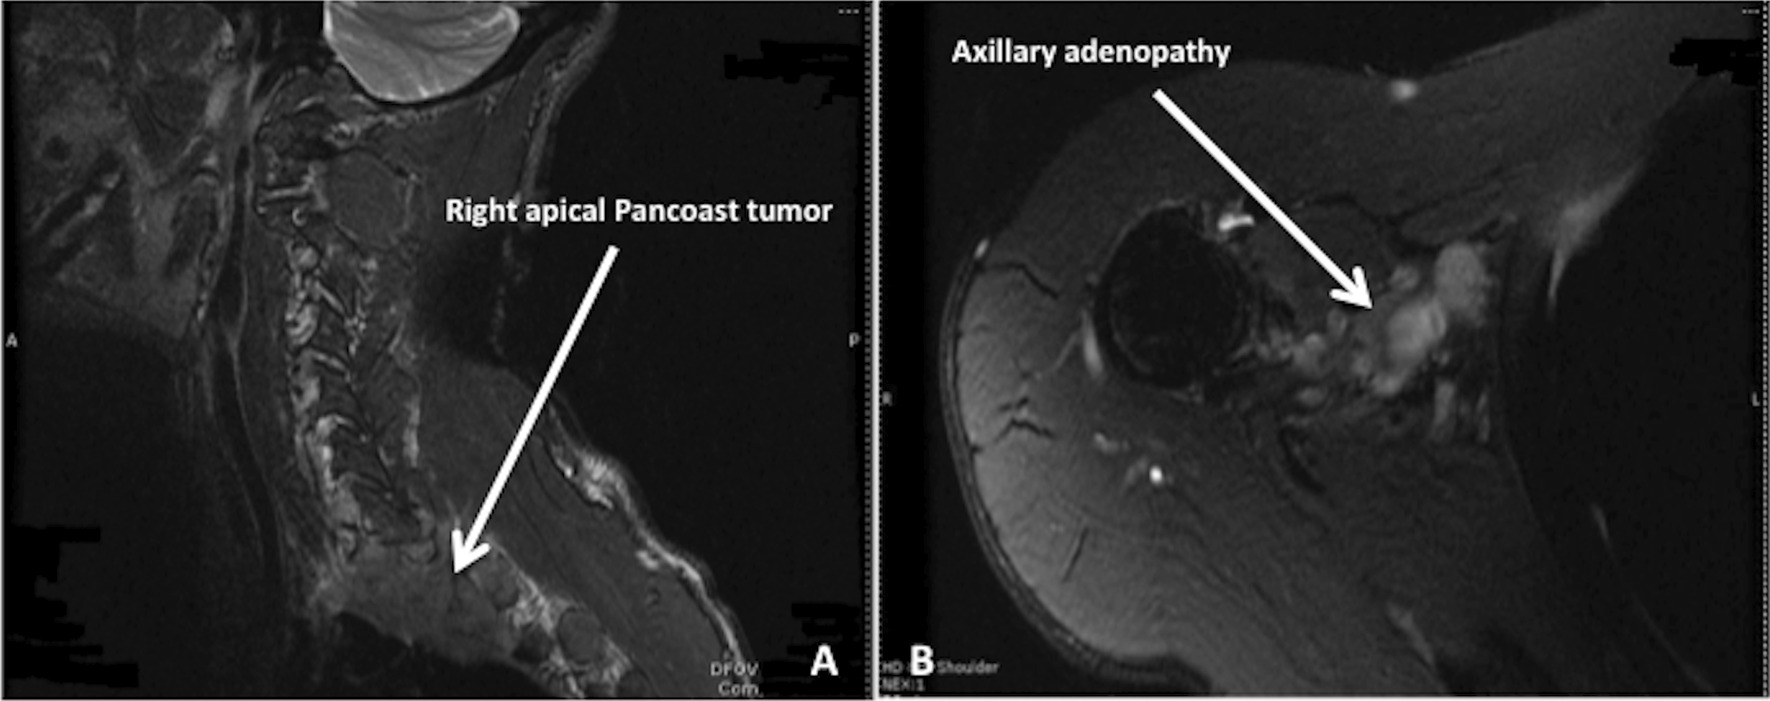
A Cervical spine MRI showing right apical mass (arrowhead) and mild cervical spondylosis. B Right shoulder MRI showing glenohumeral joint effusion (arrowhead). The rotator cuff and labrum are unremarkable
